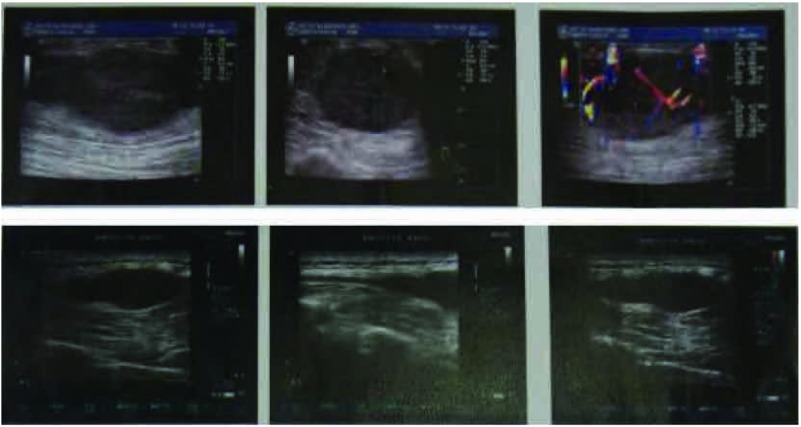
彩色超声多普勒检查示左上臂及背部软组织肿物 Color hepersound Doppler demonstrated masses in left arm and back soft tissue
